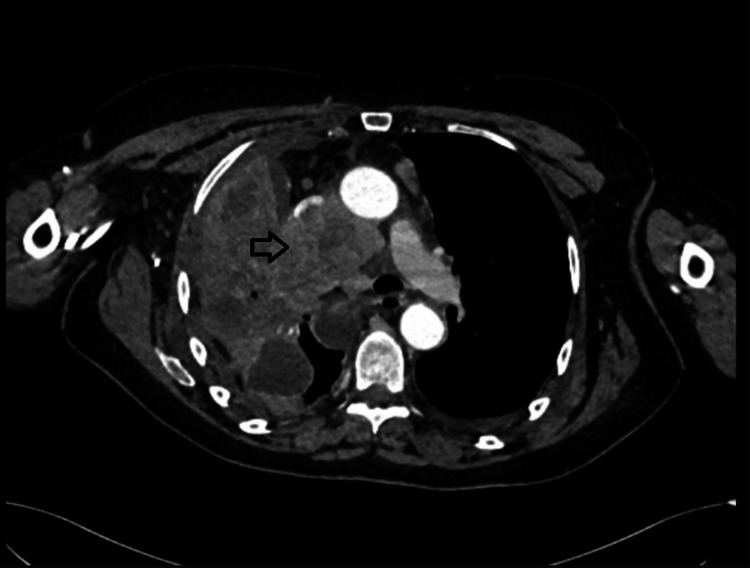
CT thorax showing a large right-sided lung mass (black arrow) with associated mediastinal adenopathy.
